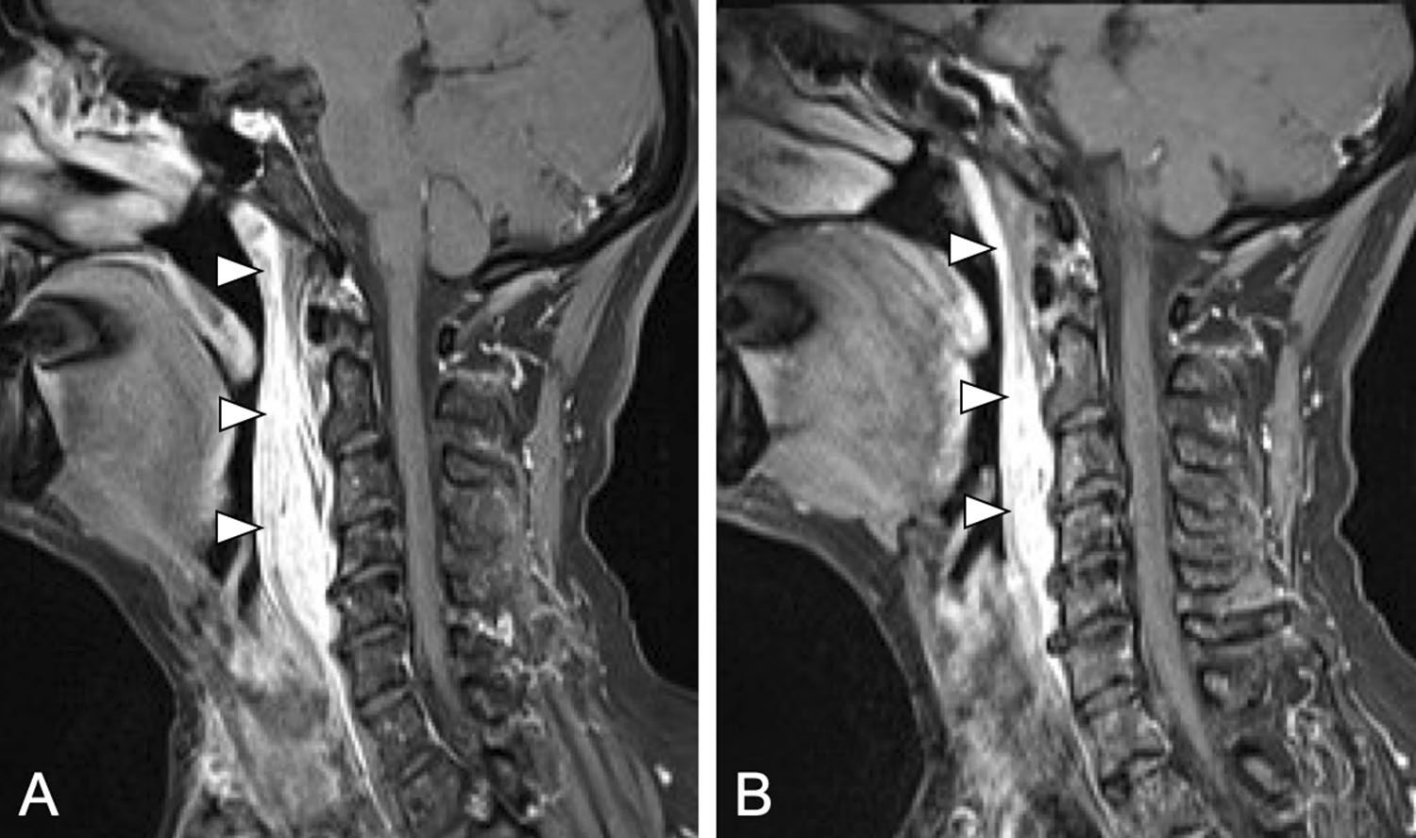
Short-term remission of MRI findings in a 55-year-old female patient (Patient 3) initially presenting at another hospital with fever and tachycardia, who was treated with oral antibiotics. During the course of treatment, she developed progressive odynophagia. On clinical presentation at our institution, symptoms had already decreased. a Sagittal T1-weighted image with fat saturation after contrast agent administration of the initial MRI performed on the day of admission with large edema in the retropharyngeal space at level C1–C5/6 (white arrowheads). RCT was diagnosed and a conservative treatment was initiated. b Sagittal T1-weighted follow-up MRI with fat saturation 8 days after initiation of the treatment showing decreased inflammatory infiltrations (white arrowheads). By that time, the patient was asymptomatic
